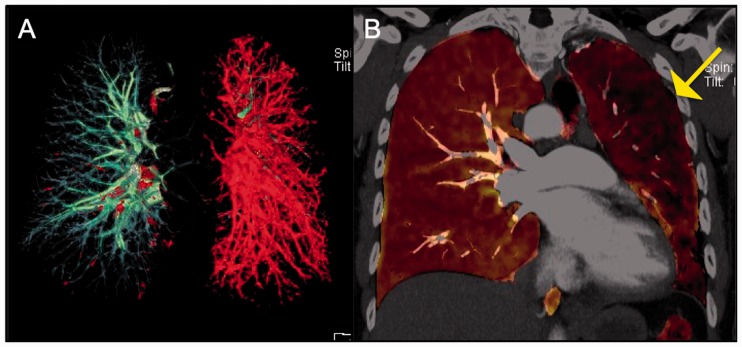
Perfused blood volume images from Dual energy CT data demonstrate
